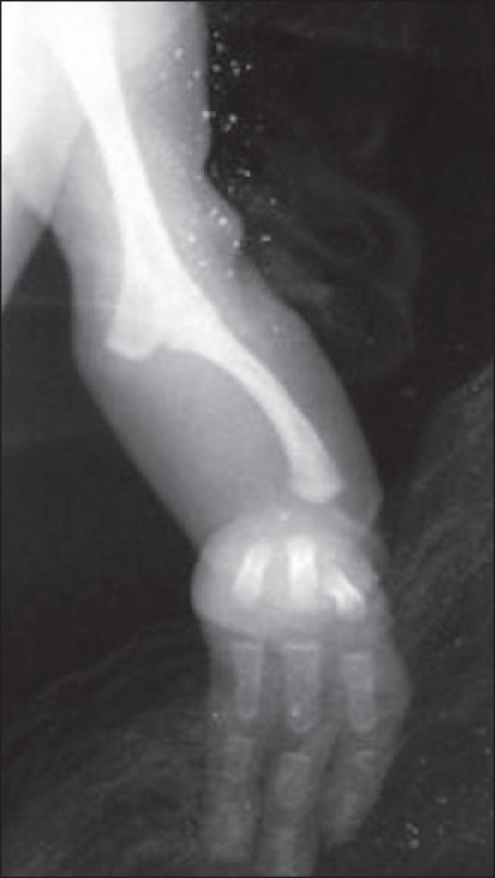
X-ray of the upper limb revealing absence of radius, bowed ulna, and humeroulnar synostosis
